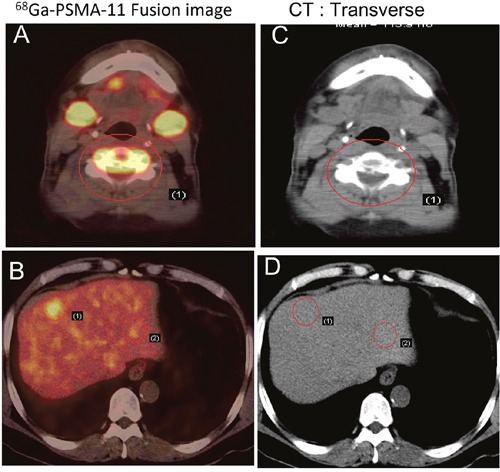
Early detection of PCa metastasis on 68Ga-PSMA-11 PET/CT images Two years after androgen-deprivation therapy, a patient with mCRPC showed elevated PSA (5.2 ng/ml) and moderate uptake in the fifth cervical vertebra on a bone scan. PSMA avid lesions were at C5 and in the liver. A, B. 68Ga-PSMA-11 PET/CT fusion image. C, D. CT image.
